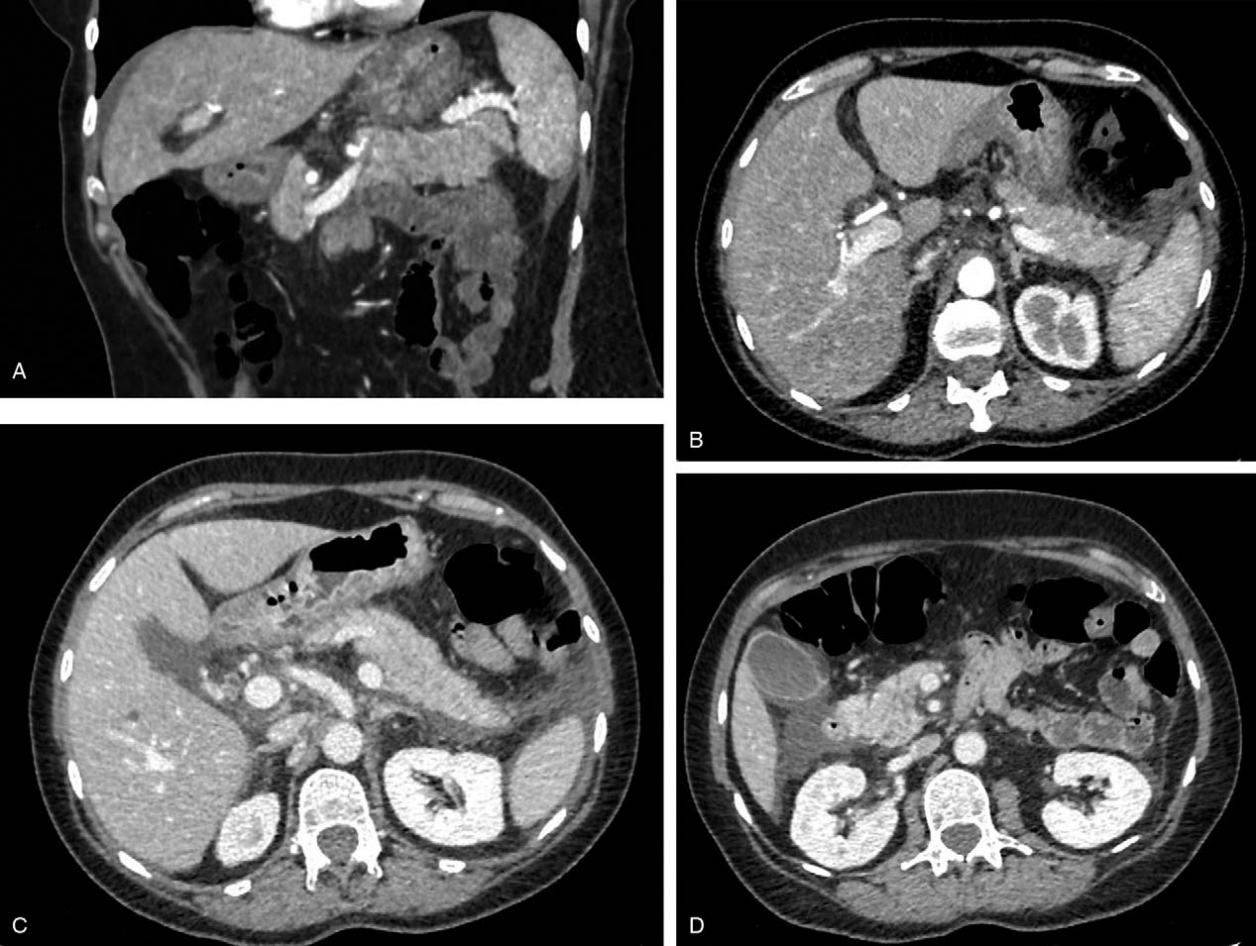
Abdominal CT: area of pancreatic parenchymal necrosis (hypodense notch of the tail of the pancreas <30%) in coronal section (A) and in axial section (B), peri-splenic necrosis casting also in front of the left anterior para-renal fascia (C), necrosis casting also in front of the right anterior para-renal fascia (D).
